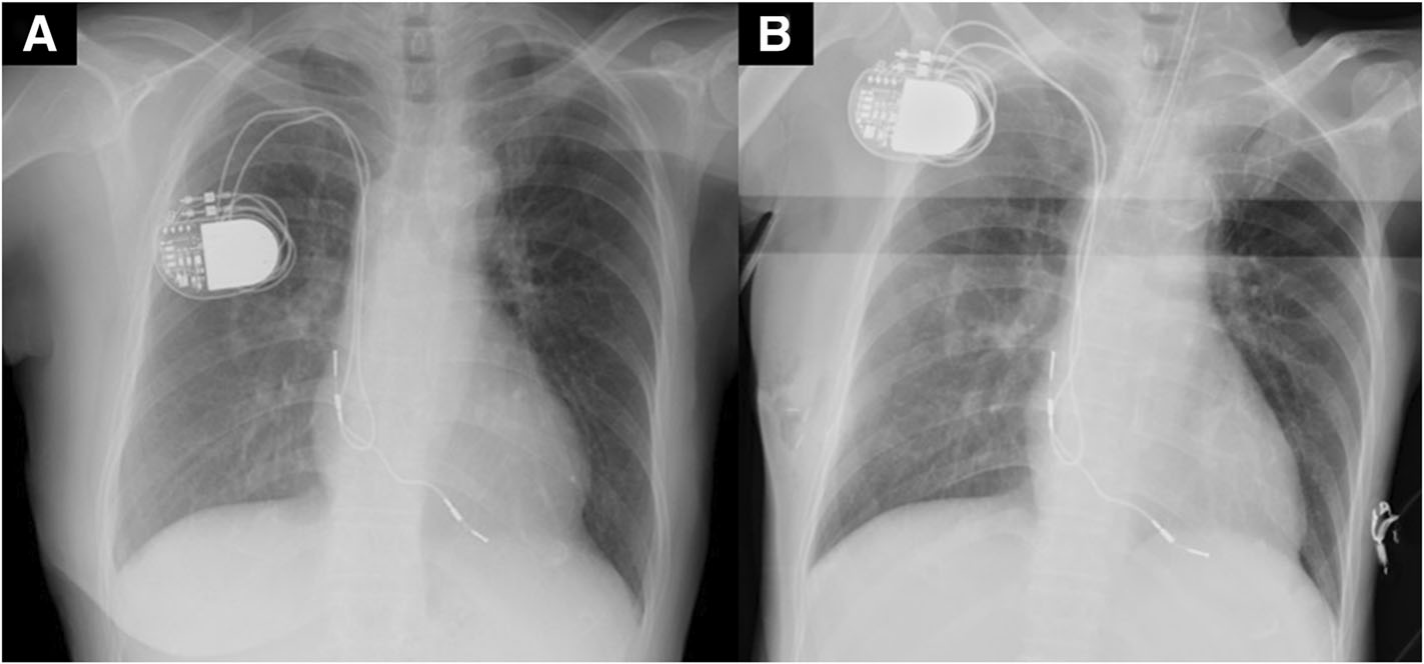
Preoperative (a) and postoperative (b) chest radiograph. Although the postoperative chest radiograph showed a slight change in generator position compared to the preoperative chest radiograph, no structural problems, including displacement of the pacing leads, perforation, cardiac dilatation, or pericardial effusion, were noted
